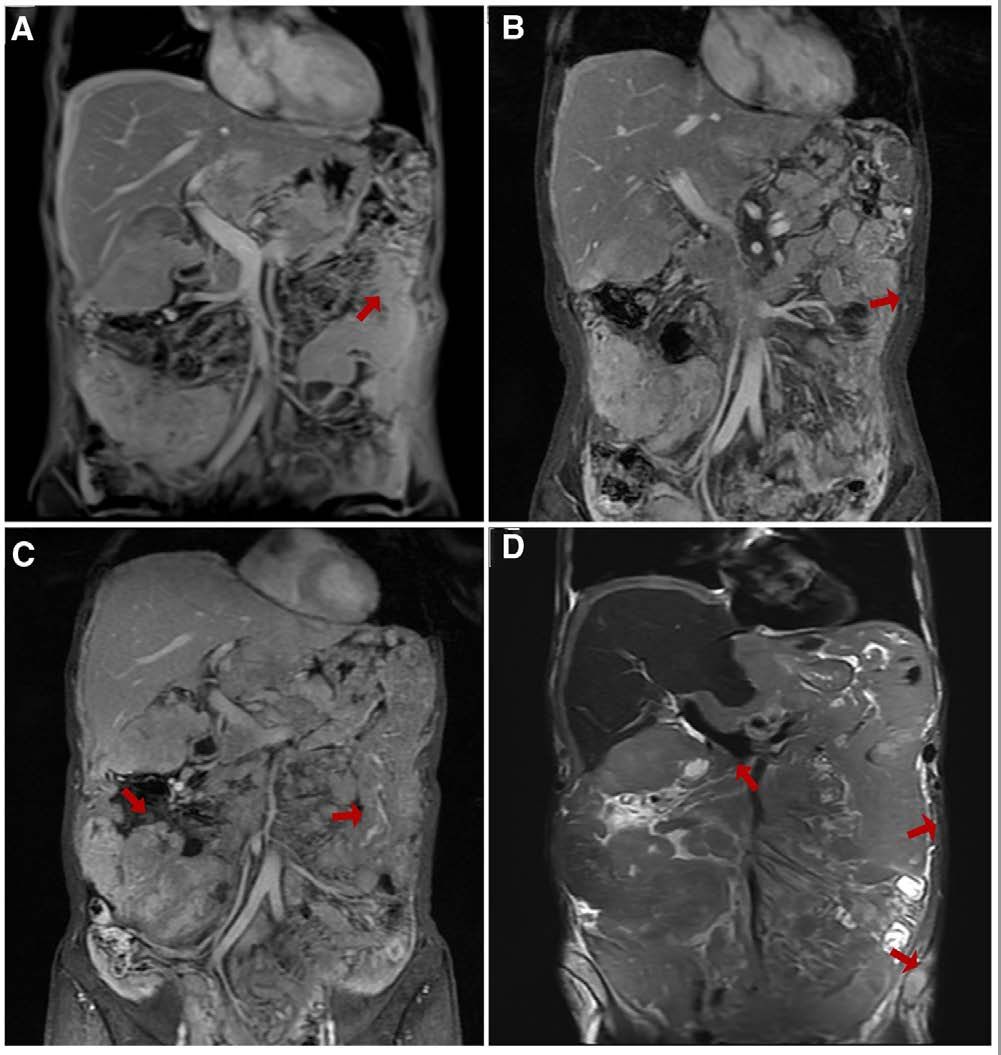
T2 the MRI of DMPM: (A) before pemetrexed plus nivolumab treatment in June 2020; (B) after 6 cycles of pemetrexed combined with nivolumab, the evaluated was SD; (C) after 12 cycles of nivolumab, the evaluated was PD, before radiotherapy; (D) After the combination of anlotinib and nivolumab in June 2020, the evaluated was PD. DMPM = diffuse malignant peritoneal mesothelioma, PD = progressive disease, SD = stable disease.
